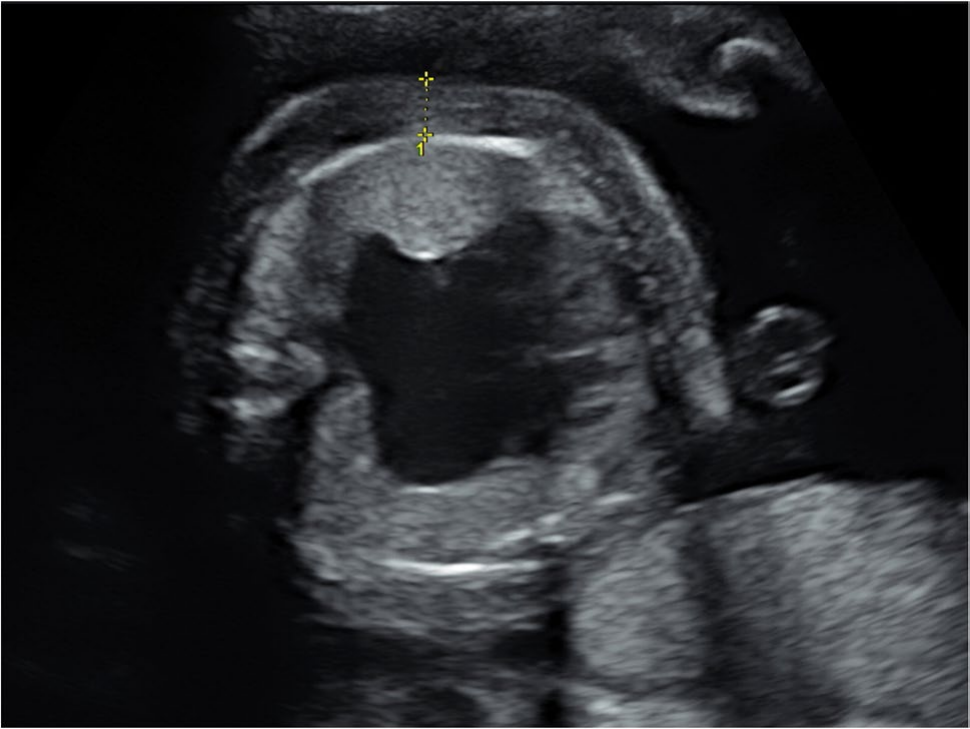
Case 1 at 24 + 1 weeks of gestation, skin edema measuring 7.4 mm, CVPS 5 points (skin edema: 0 points, mild cardiomegaly (cardiothoracic area ratio = 0.45): 1 point, mitral valve regurgitation: 0 points, normal arterial Doppler flow velocimetry: 2 points, normal venous Doppler flow velocimetry: 2 points)
